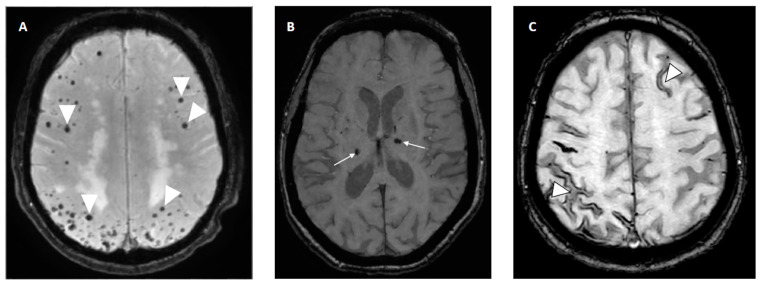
Radiologic bleeding markers on susceptibility weighted imaging (SWI) on MRI. (A) Multiple lobar cerebral microbleeds (CMBs) in patient with cerebral amyloid angiopathy (CAA). (B) Deep CMBs related to hypertension. (C) Cortical superficial siderosis (cSS) in frontal left hemisphere and posterior right sulci in patient with CAA.
